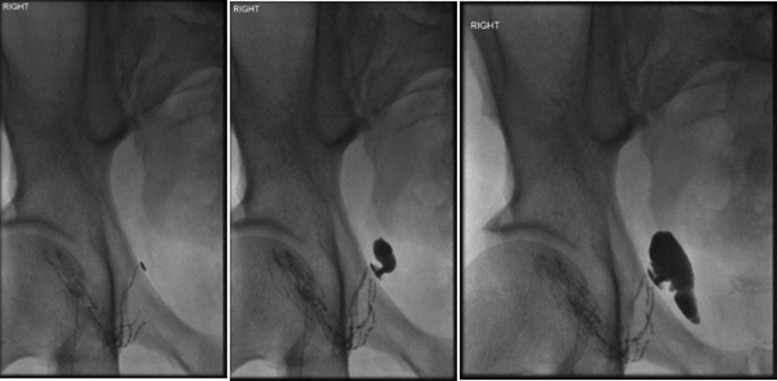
Lymphangiogram; Ultrasound-guided right superficial lymph node puncture. Lipiodol was infused. Leak identified at level of distal external iliac vessels just superior to the superior pubic ramus.
